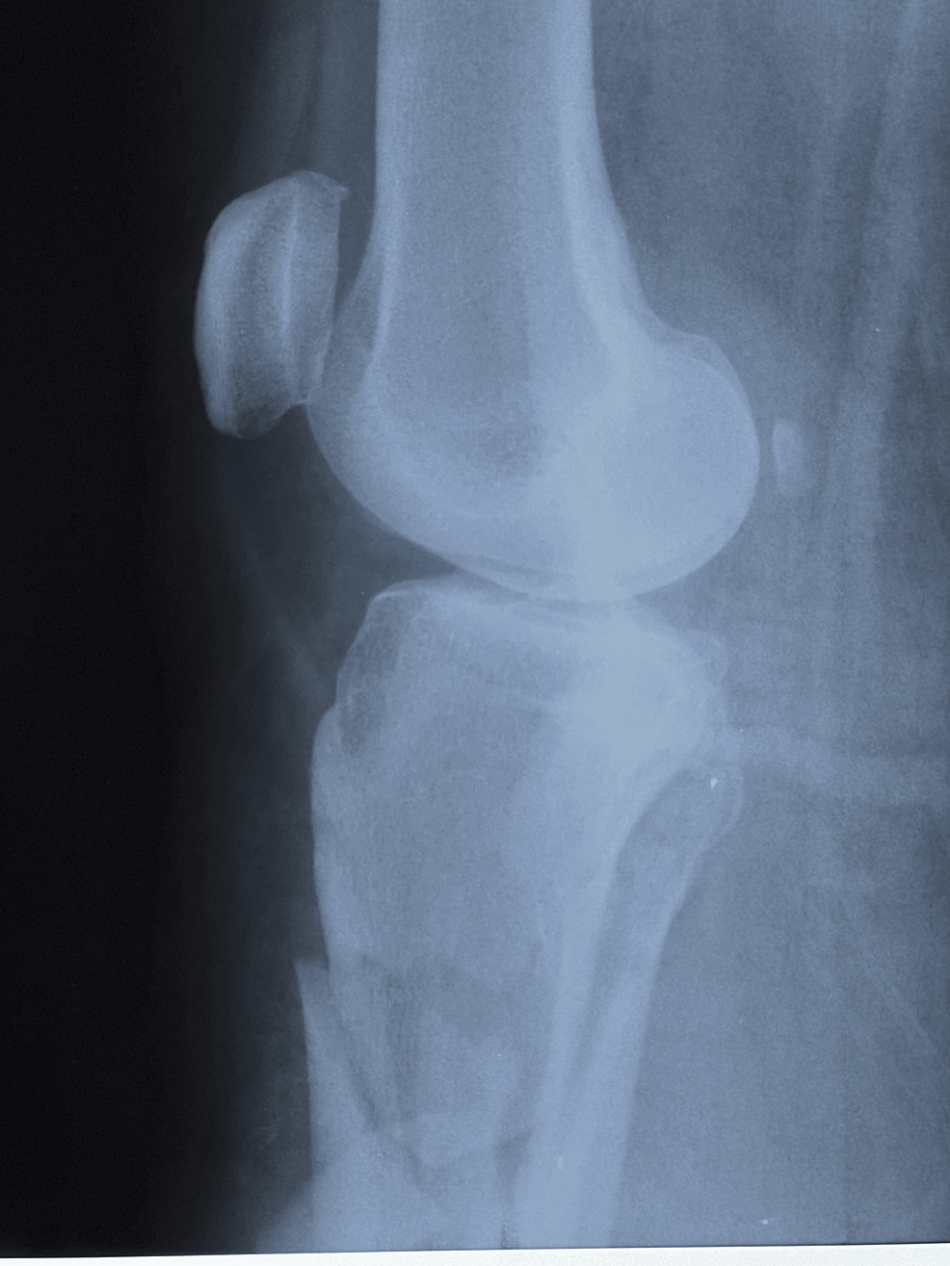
Lateral X-ray of the right knee and proximal right leg showing tibial metaphyseal comminuted fracture and fracture of the proximal fibula
